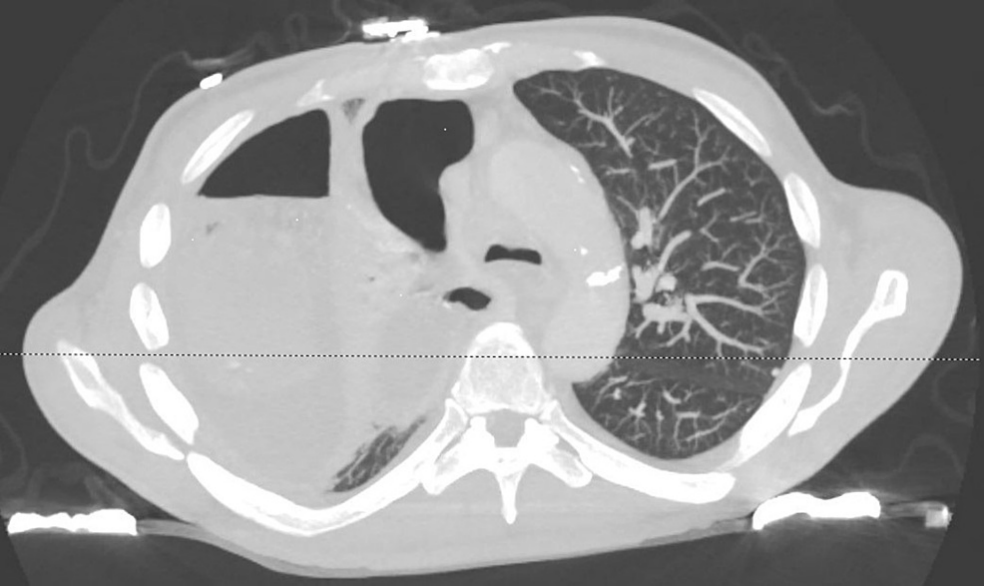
Transverse view of CT chest showing near-complete opacification of the right upper, middle, and lower lobes
